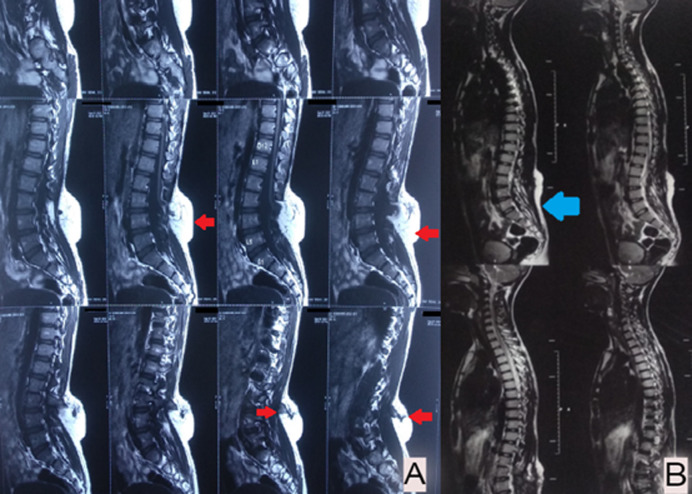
A) red arrow indicates lipomyelocele at lumbosacral region; B) post-operative MRI, blue arrow indicates thickened filum terminale and tethering at L2-L3 level
